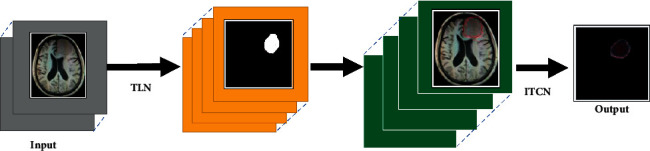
The MRI brain tumor convolutional network structure based on the CNN algorithm.
